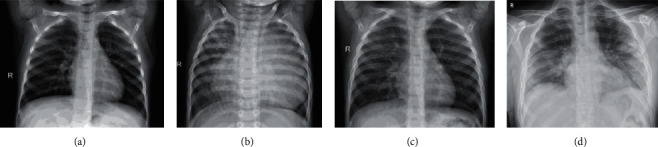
Example chest X-ray image of: (a) Healthy. (b) Bacteremia. (c) Viral pneumonia. (d) COVID-19 viral infection.
